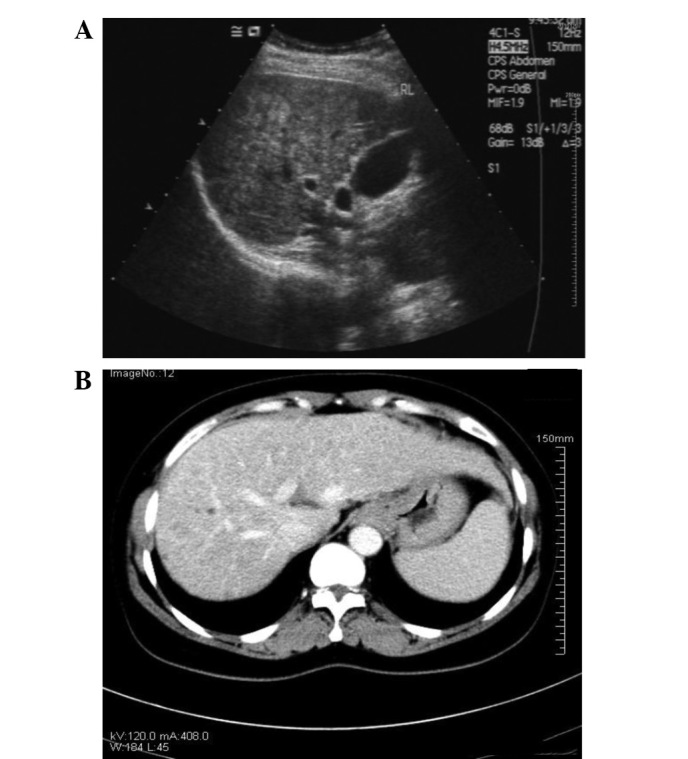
(A) B-mode ultrasound indicating a liver echo change as a solid lump in the left liver; CEUS was consistent with the diagnosis of small hepatocellular carcinoma (HCC). (B) Abdominal enhanced CT indicating multiple space-occupying lesions in the liver. CEUS, contrast-enhanced ultrasound.
